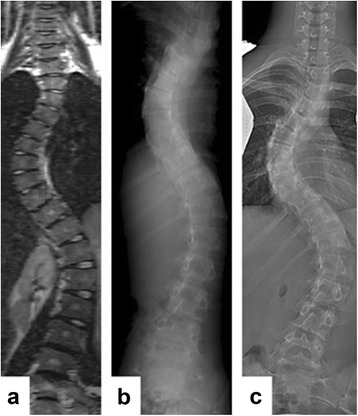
On the MRI and CT images, the main thoracic and (thoraco)lumbar Cobb angle, thoracic kyphosis, and lumbar lordosis were measured using the same technique as for the conventional radiographs on the image where the curve and endplates were best visible by using the multiplanar reconstruction (MPR, a) for the MRI and the digitally reconstructed radiograph (b) for the CT scan. c The conventional X-ray
